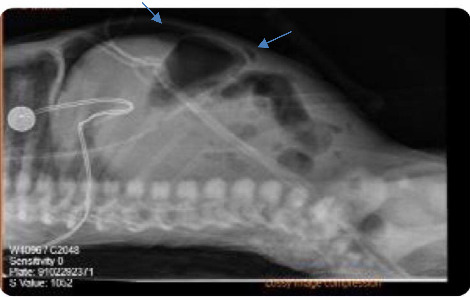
Supine lateral abdominal X-ray showing free intraperitoneal air (blue arrows) consistent with bowel wall perforation.
